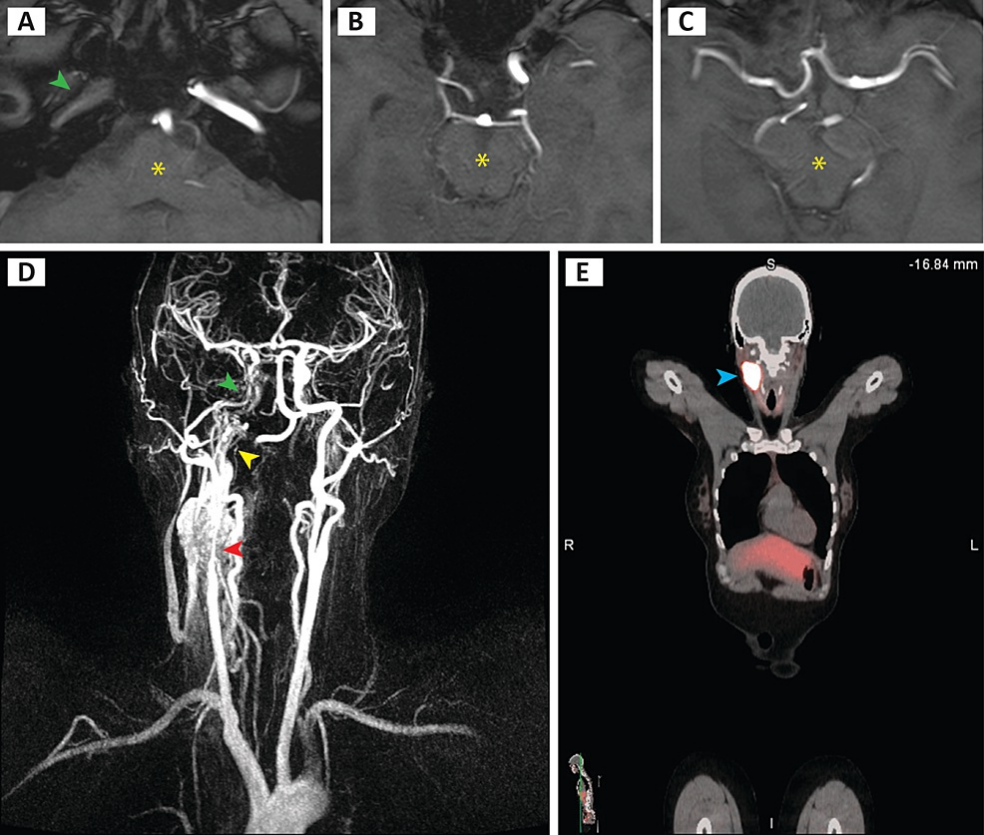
Pre-operative imaging. (A-C) MRI angiography transverse views of the petrous segment and terminal branches of ICA, with yellow asterisk depicting the medulla (A), lower midbrain (B), and upper midbrain (C). D) 3D reconstruction of MRI angiography coronal view. E) PET-CT coronal view. ICA, internal carotid artery; PET-CT, positron emission tomography-computed tomography
